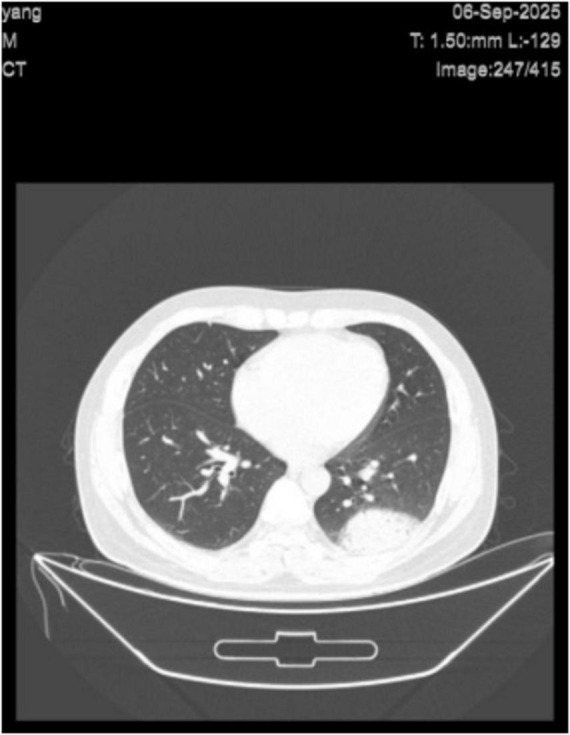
Axial cross-sectional CT scan of the chest clearly demonstrates the lungs, heart, and chest wall. A mass-like high-density opacity with surrounding haziness is observed in the left lower lobe, with adjacent costal pleural thickening, suggestive of infection. Minimal inflammation is also seen in the right lower lobe.
